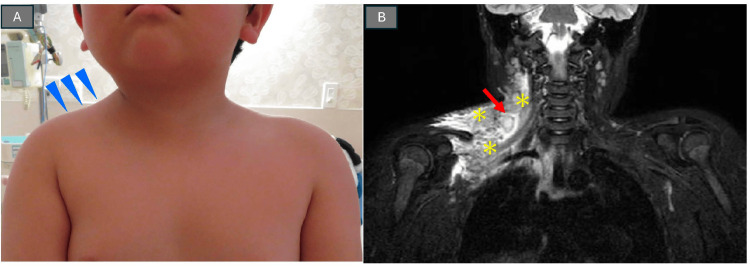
Images of an asymmetrical shoulder in a five-year-old boy suggestive of supraclavicular lymphadenitis caused by an insect bite on the ipsilateral distal extremity (A) Anterior chest photograph showing right shoulder swelling and the resulting “asymmetrical shoulder” appearance. (B) Coronal STIR-MRI imaging corresponding to (A), demonstrating an enlarged, hyperintense right supraclavicular lymph node (red arrow), with surrounding subcutaneous edema (asterisks), leading to the shoulder swelling.
